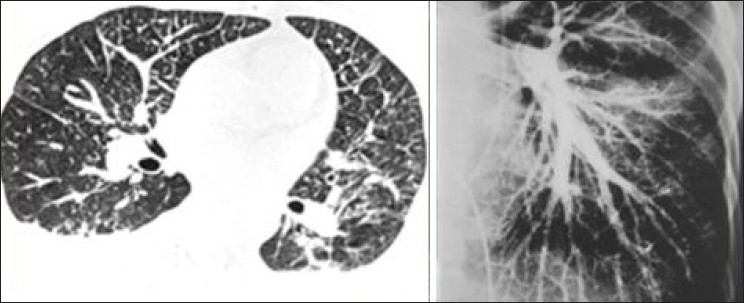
Axial contrast enhanced CT (right) and a pulmonary angiogram (left) show dilated pulmonary vasculature in a 53-year-old man with CLD associated with HPS
